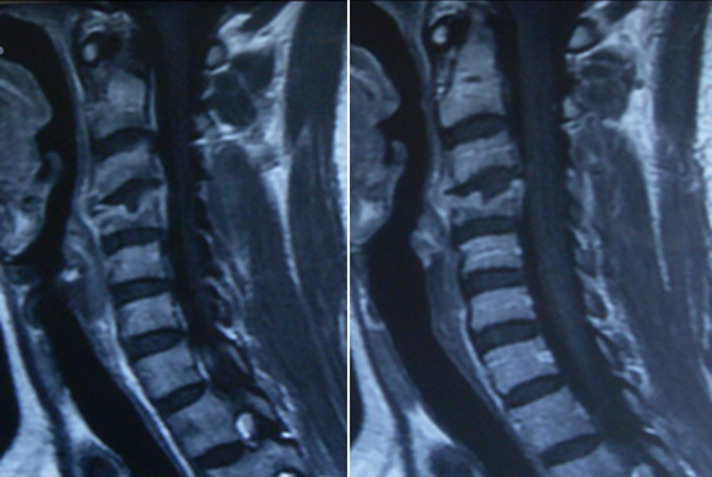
magnetic resonance imaging revealed a compression of the C4 body with epiduritis, or spinal epidural abscess, and infiltration of the paravertebral soft tissues, suggesting primarily an infectious origin
